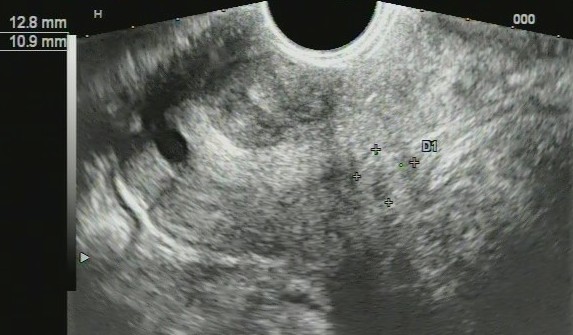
Ultrasound scans of cervical dysplasia. (A) Mild cervical dysplasia. (B) Severe cervical dysplasia. (C) Sonoelastography reveals stiff areas in severe dysplasia. (D) Cervical nodule. (E) Sonoelastography reveals stiff nodule in severe dysplasia.
